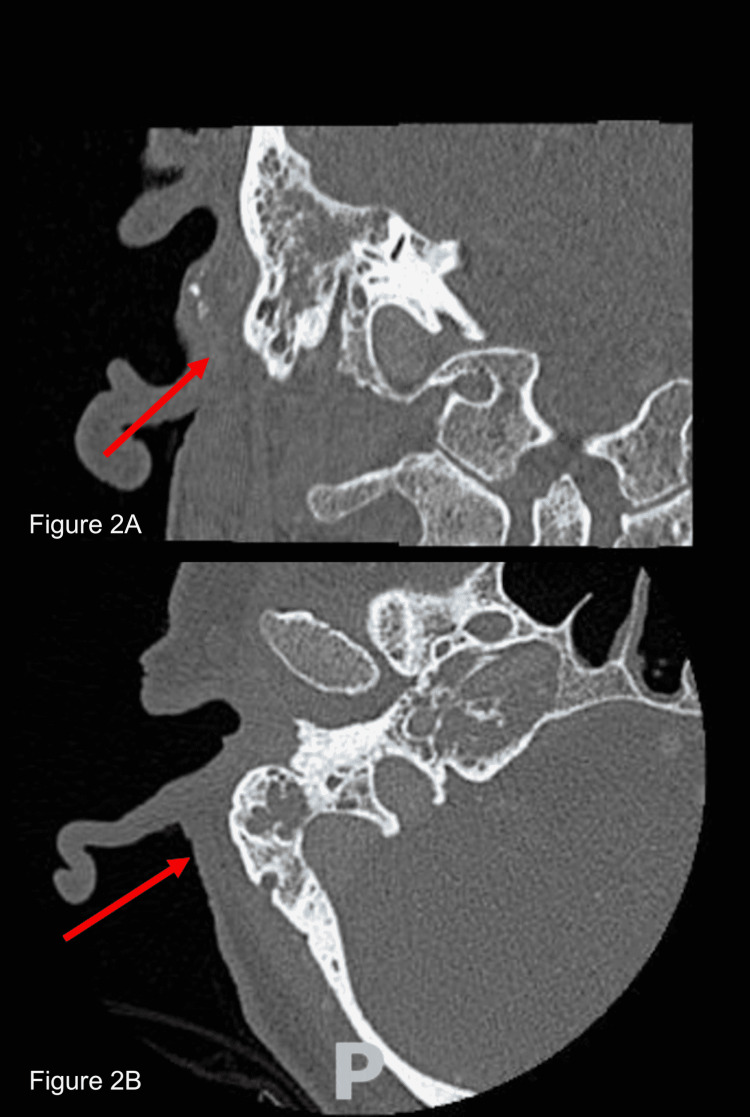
Computed tomography scan of the orbits with intravenous contrast (A) Coronal bone view; (B) axial bone view, both depicting right otomastoiditis with mastoid air cell septal erosion (red arrows).
